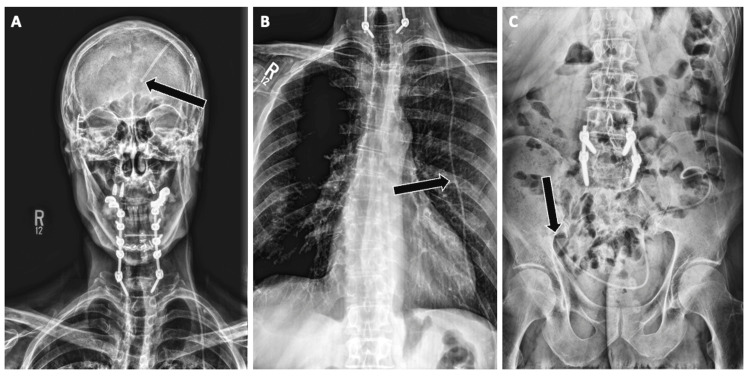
Radiographic Series Demonstrating Appropriate Ventriculoperitoneal (VP) Shunt Placement Radiographic evaluation of a properly positioned VP shunt. (A) Head and neck radiograph shows the proximal catheter tip within the lateral ventricle and its course descending through the neck (arrow). (B) Chest radiograph demonstrates the shunt traversing the mediastinum toward the abdomen (arrow). (C) Pelvic radiograph shows the distal catheter tip coiled appropriately within the peritoneal cavity (arrow). Sequential imaging across these regions is essential to confirm shunt integrity and appropriate positioning from ventricle to peritoneum.
